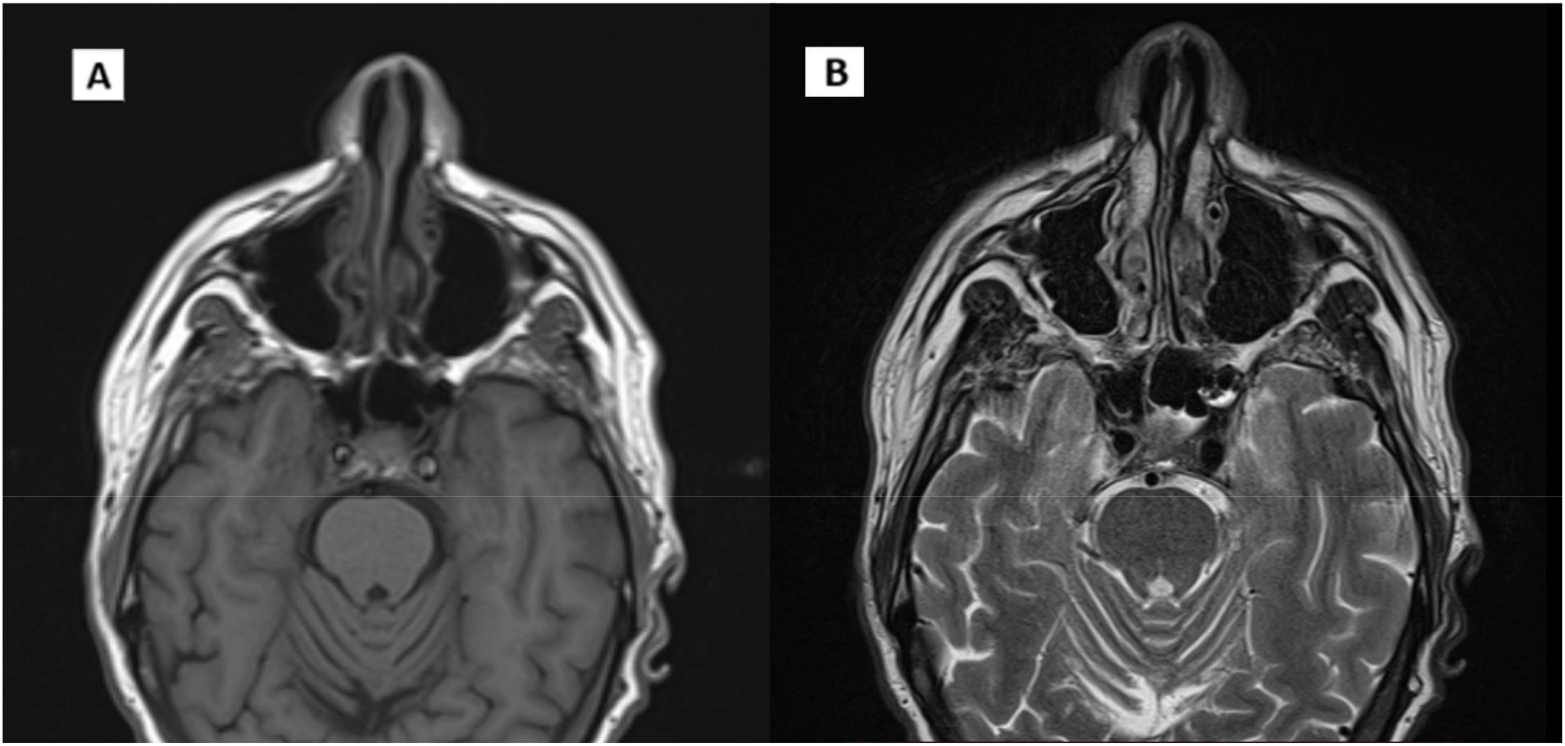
Post IO MRI scans. (A) T1 axial scan. (B) T2 axial scan.
